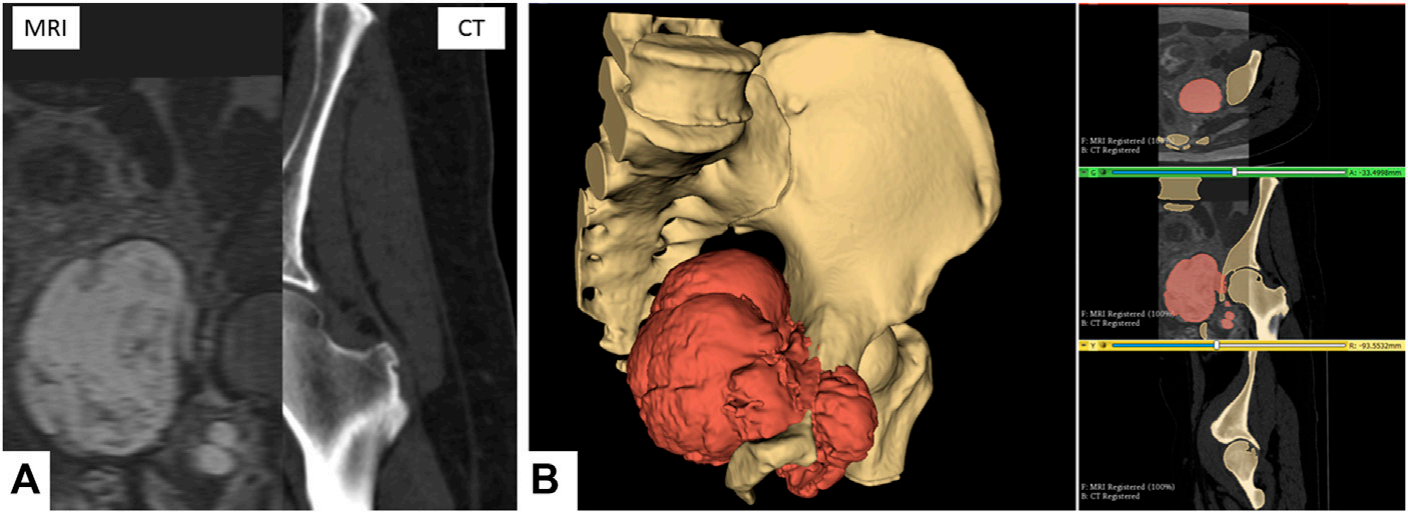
Multimodal segmentation. (A) CT and MRI images, non-rigid B-Spline registration (3D Slicer’s Elastix). (B) Multimodal segmentation using Watershed (right) and 3D reconstruction (left) of the bone tissues and tumor. Scale = 50 mm.
